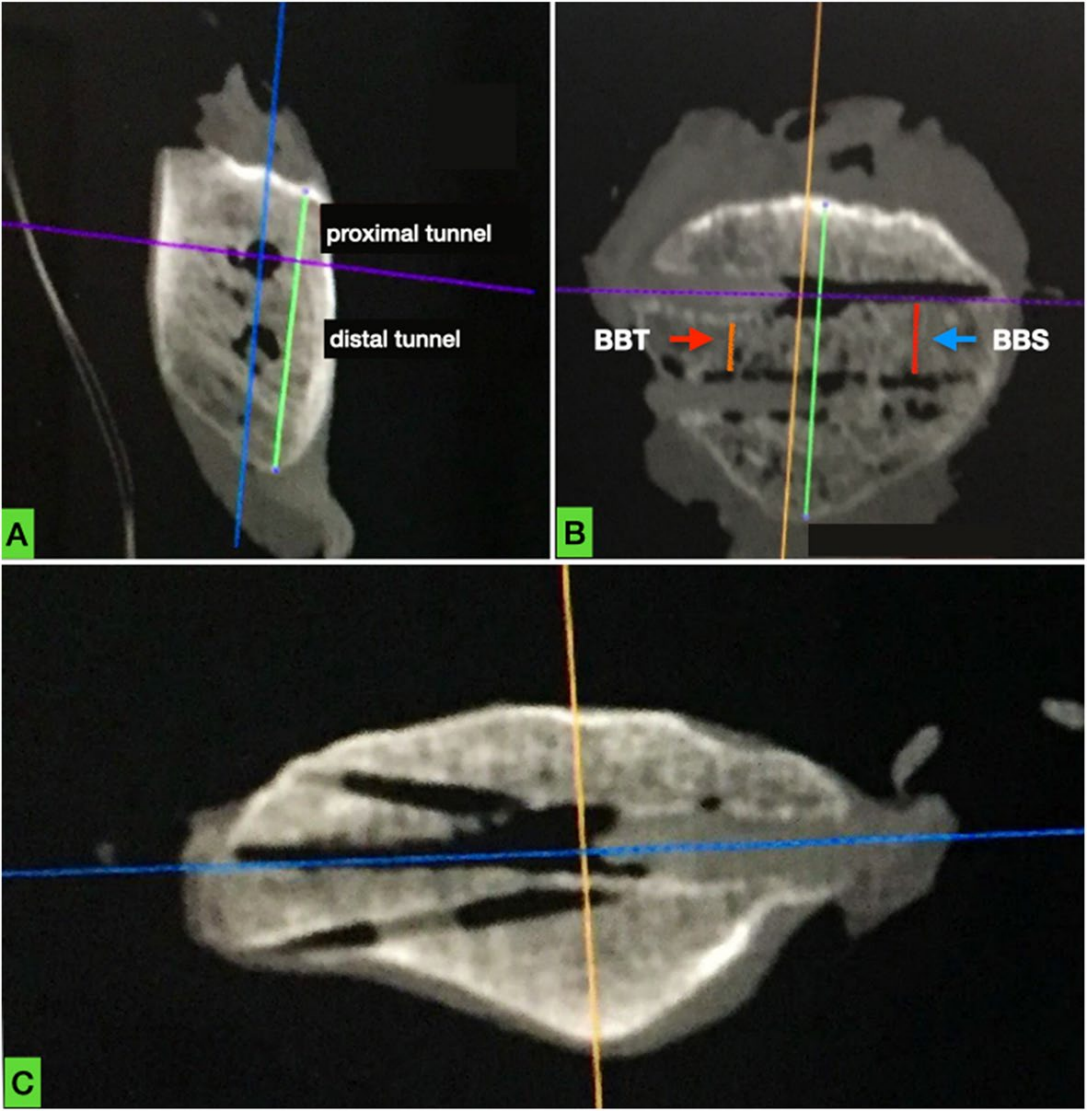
Sagittal, frontal and axial CT section of patella #7 postoperatively. Tunnel placement in a and b appears adequate. However, in c, multiple attempts (with breaching of the articular surface and the anterior cortex) made by the surgeon for proximal tunnel placement are revealed. Blue arrow in b points at the postoperative bone bridge (red line) between transosseous sutures (BBS). Red arrow in b points at the postoperative bone bridge (orange line) between tunnels (BBT)
